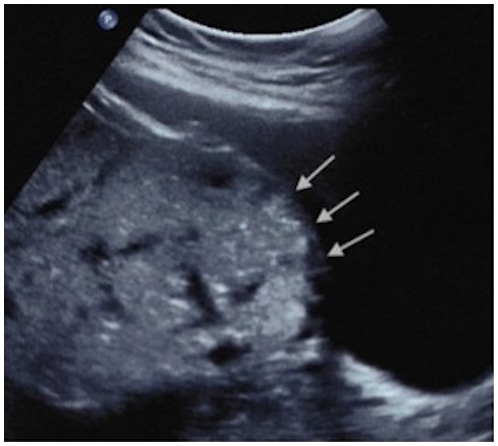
Uterine bulging and disruption of the hyperechogenic uterine serosa-bladder wall interface on ultrasonography.
